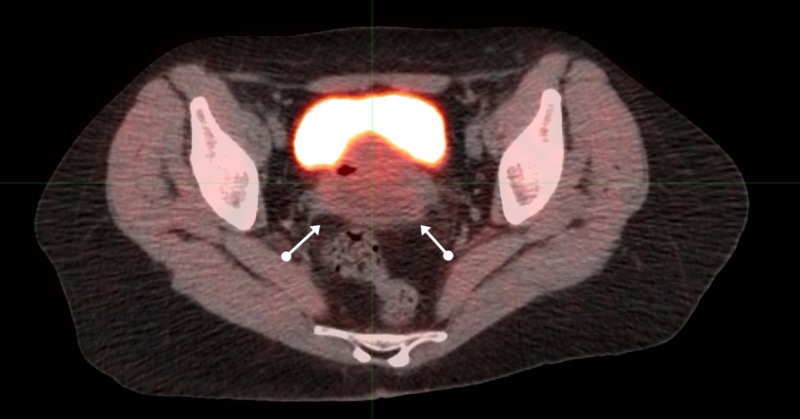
Sequential axial PET/CT images of pelvic malignancy: proximal positron emission tomography-computed tomography: PET/CT
